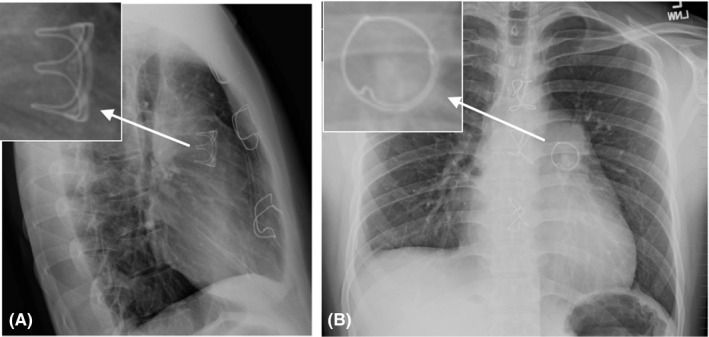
Postoperative chest radiograph in lateral (A) and PA view (B), showing the 25 mm Inspiris valve in place
